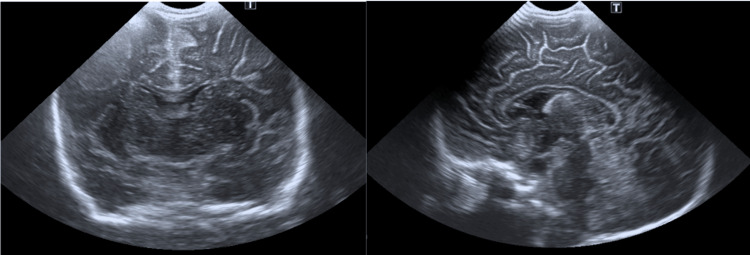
Transfontanellar ultrasound image - third coronal plane (left) and median sagittal plane (right).
